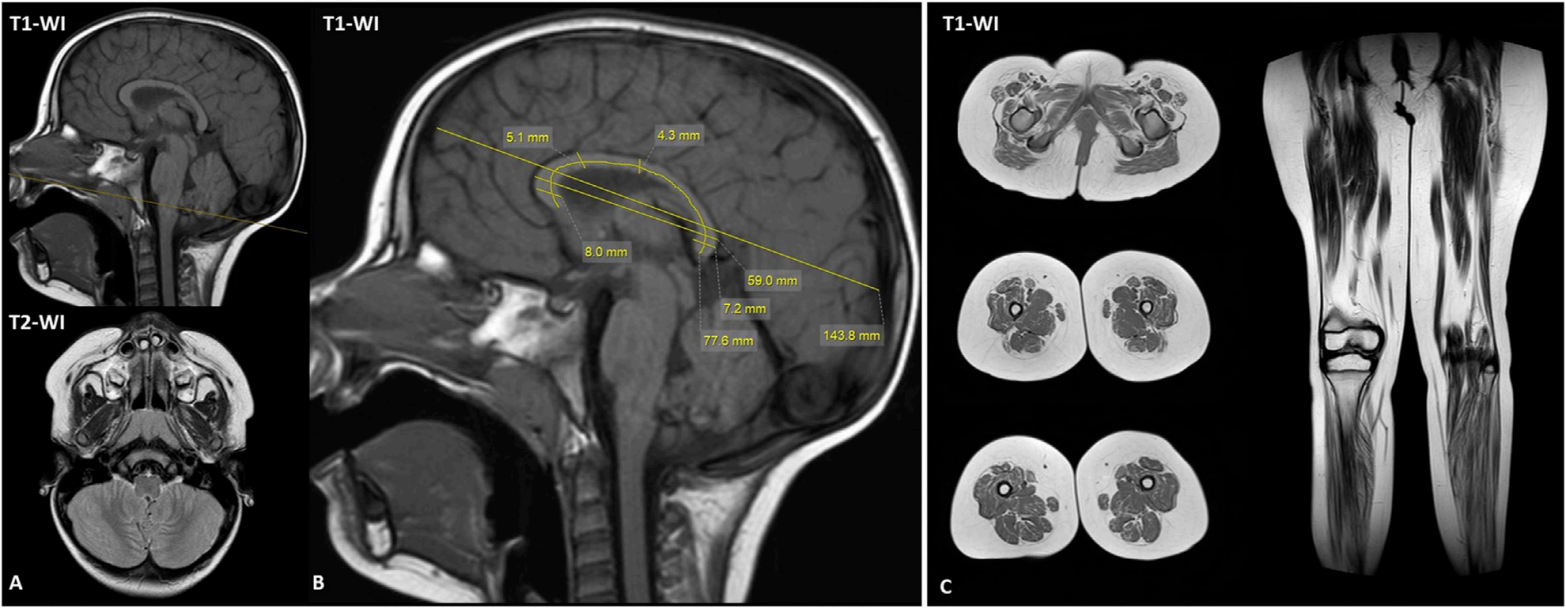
Magnetic resonance imaging of brain and lower limb muscles. (A) Brain MRI shows no structural brain abnormalities. (B) The brain MRI demonstrated normal range corpus callosum measurements for 3-year-old female proband. (C) The lower limb muscle MRI revealed diffuse fatty infiltration predominant in lower legs. T1-WI, T1 weighted image; T2-WI, T2 weighted image.
